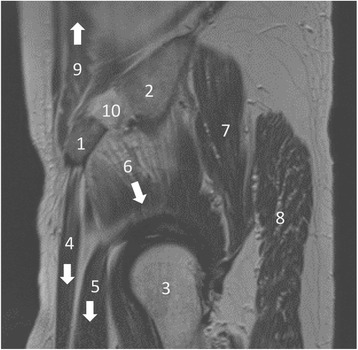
MRI of a pelvis and the hip joint in T2 sagittal reconstruction: 1 = ASIS, 2 = iliac crest, 3 = femoral head, 4 = sartorius muscle, 5 = femoral rectus muscle, 6–8 = minor, medium, and greater gluteal muscles, 9 = abdominal muscle, 10 = location of bone graft harvesting. The arrows show the traction of muscle forces that provoke a fatigue fracture of the ASIS [13]
